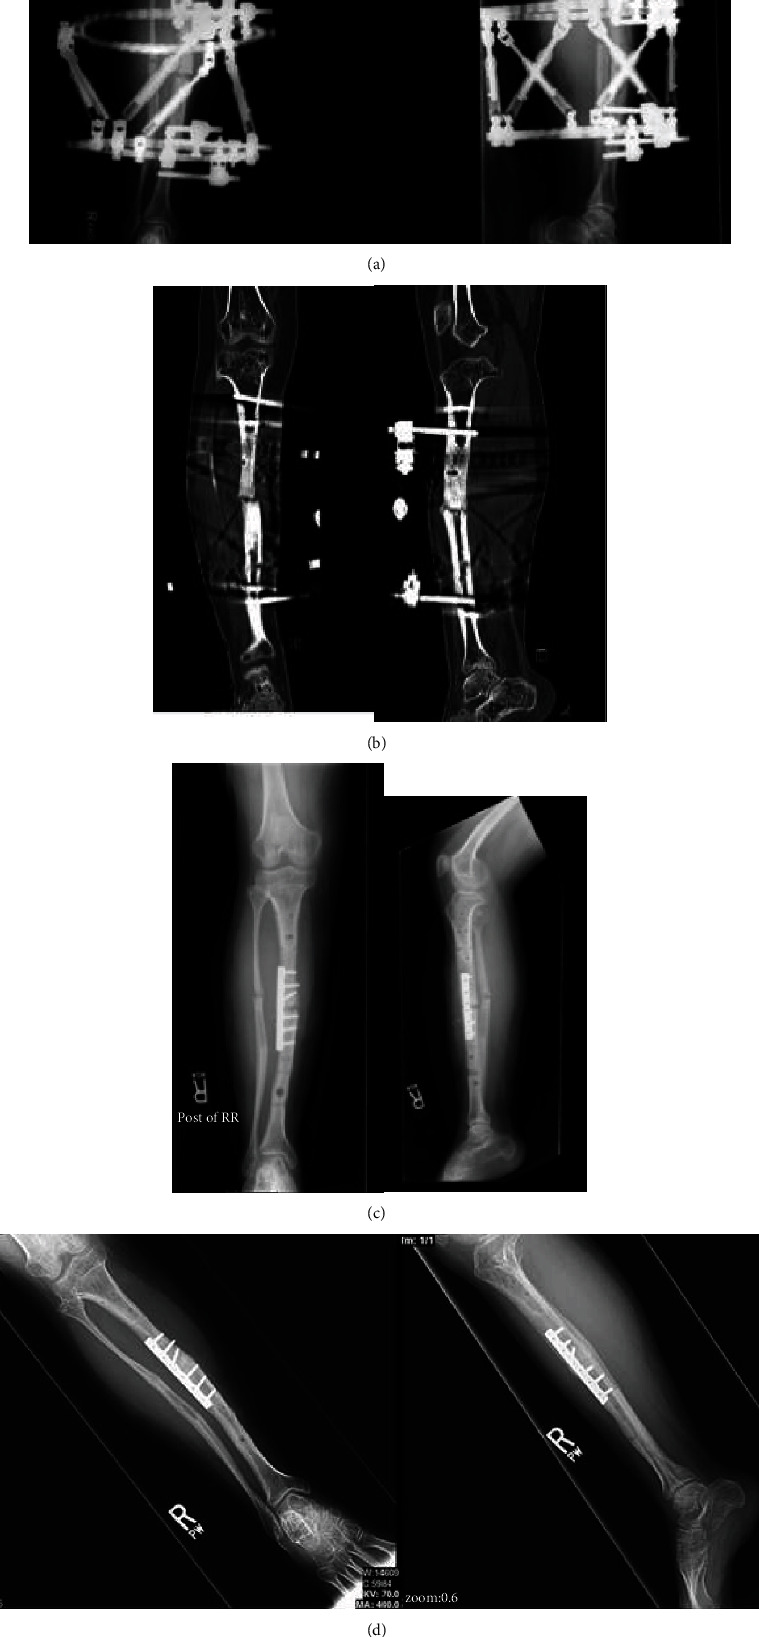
18-year-old female with achondroplasia who underwent a varus-producing diaphyseal osteotomy for valgus deformity of the tibia. (a) Anteroposterior and lateral radiographs demonstrating nonunion of diaphyseal osteotomy following Taylor spatial frame application for gradual corrective program. (b) Coronal and sagittal computed tomography reconstructions confirming nonunion of diaphyseal osteotomy despite completion of the program. (c) Revision open reduction and internal fixation with 3.5 mm plate 6 months following index frame application. (d) Radiographs obtained at 3 months following revision demonstrating union of the tibial osteotomy with no residual deformity.
